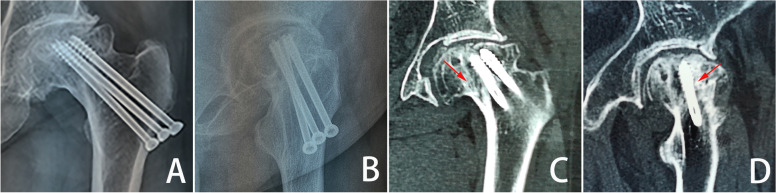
Radiographic data of a clinical femoral neck fracture case. A Coronal X-ray; B sagittal X-ray; C coronal CT; D sagittal CT. In the Figures, red arrows represent the high-density shadow around the cannulated screws
